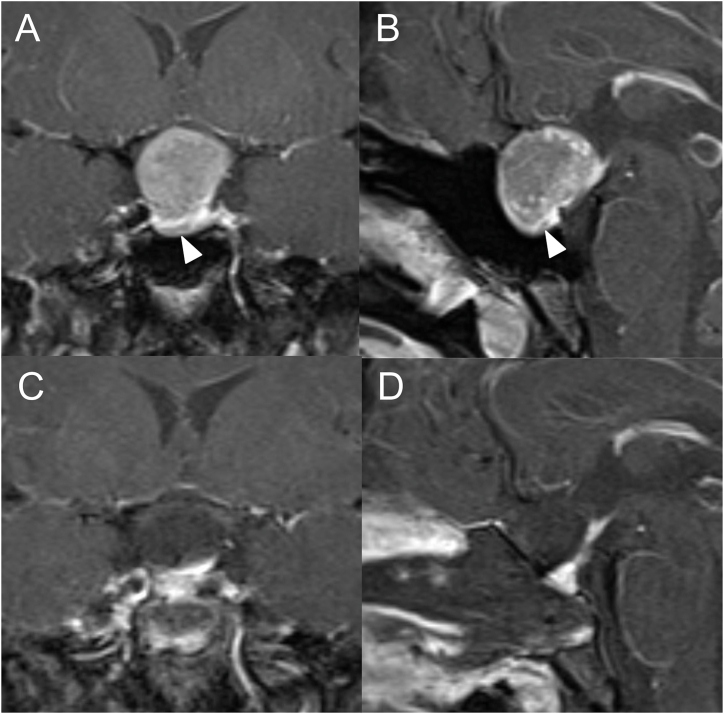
Representative Case 2: A 25-year-old woman with ectopic suprasellar GH-producing pituitary neuroendocrine tumor. Preoperative GH level was 17.41 ng/mL and IGF-1 level was 725 ng/mL (standard range: 147–358 ng/mL). Preoperative coronal (A) and sagittal (B) T1-weighted MR images after injection of gadolinium showing a tumor with maximum diameter of 20.2 mm, the normal pituitary gland below the tumor, and bottom of the sella (arrowhead). Postoperative coronal (C) and sagittal (D) T1-weighted MR images after injection of gadolinium showing complete removal of the tumor. Basal GH level was 0.38 ng/mL and IGF-1 level was 172 ng/mL, and remission was achieved after surgery.
